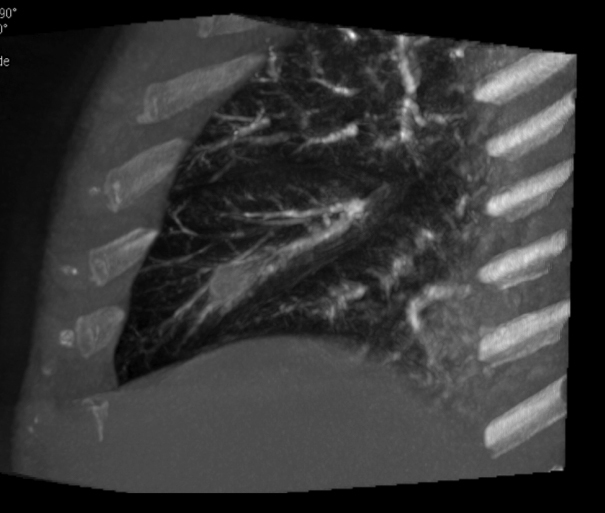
Coronal view of cone-beam CT angiography documenting the pseudoaneurysm.
